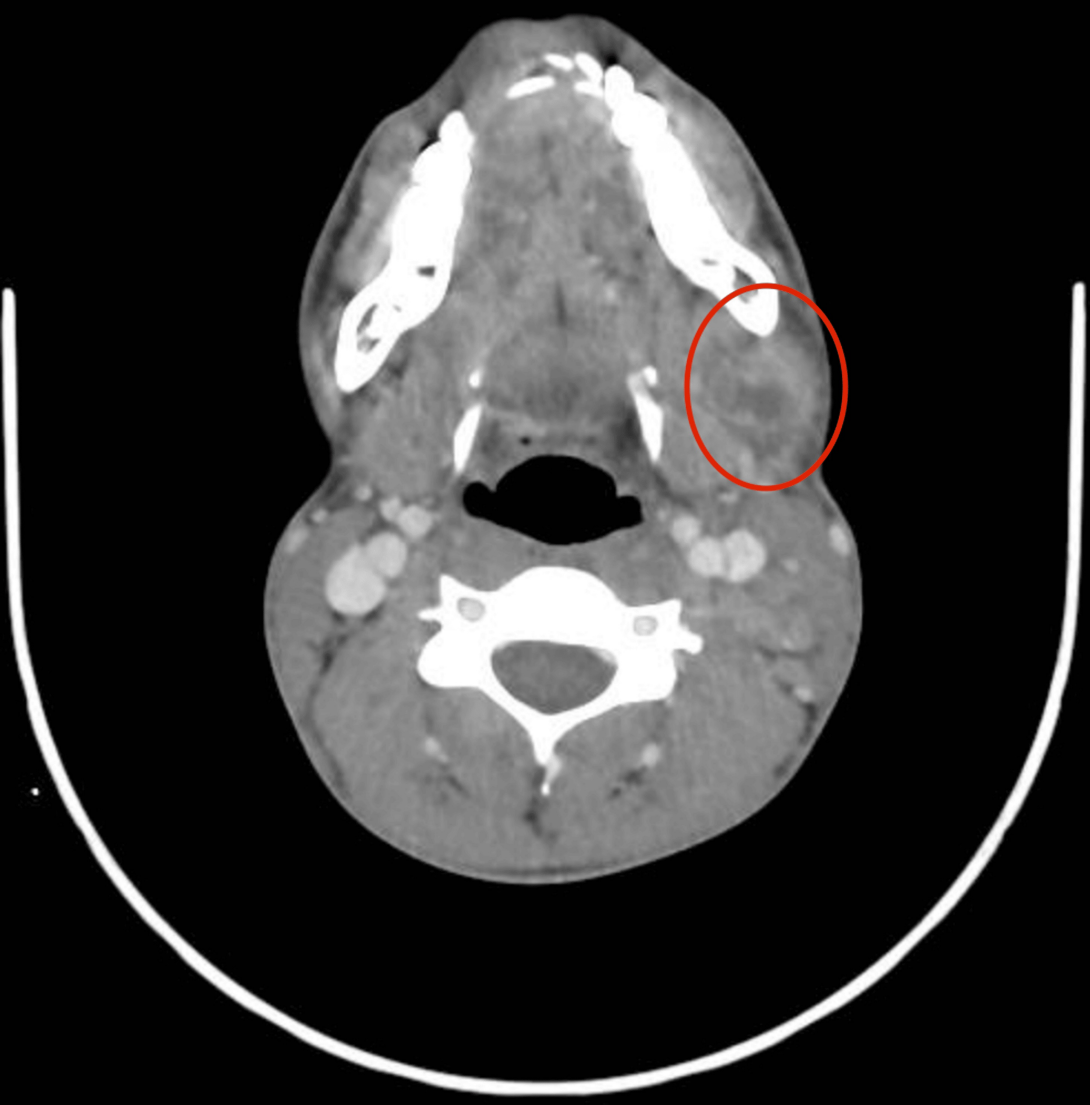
Contrast‑enhanced CT of the neck showing a necrotic lymph node. Axial CT image of the neck demonstrates a necrotic lymph node (20 × 17 mm) in the left submandibular region, circled in red.
